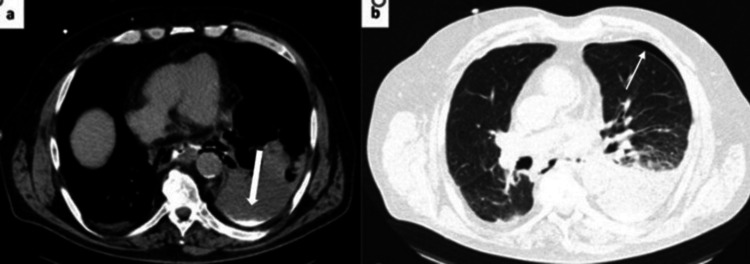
(a) CT scan with oral contrast, which demonstrates in the topography of the lower third of the esophagus, leakage of contrast material into the left pleural space, associated with ipsilateral pleural effusion, in relation to esophageal rupture (thick arrow); (b) Presence of a thin lamella of pneumothorax on the left (thin arrow). CT, Computed Tomography
